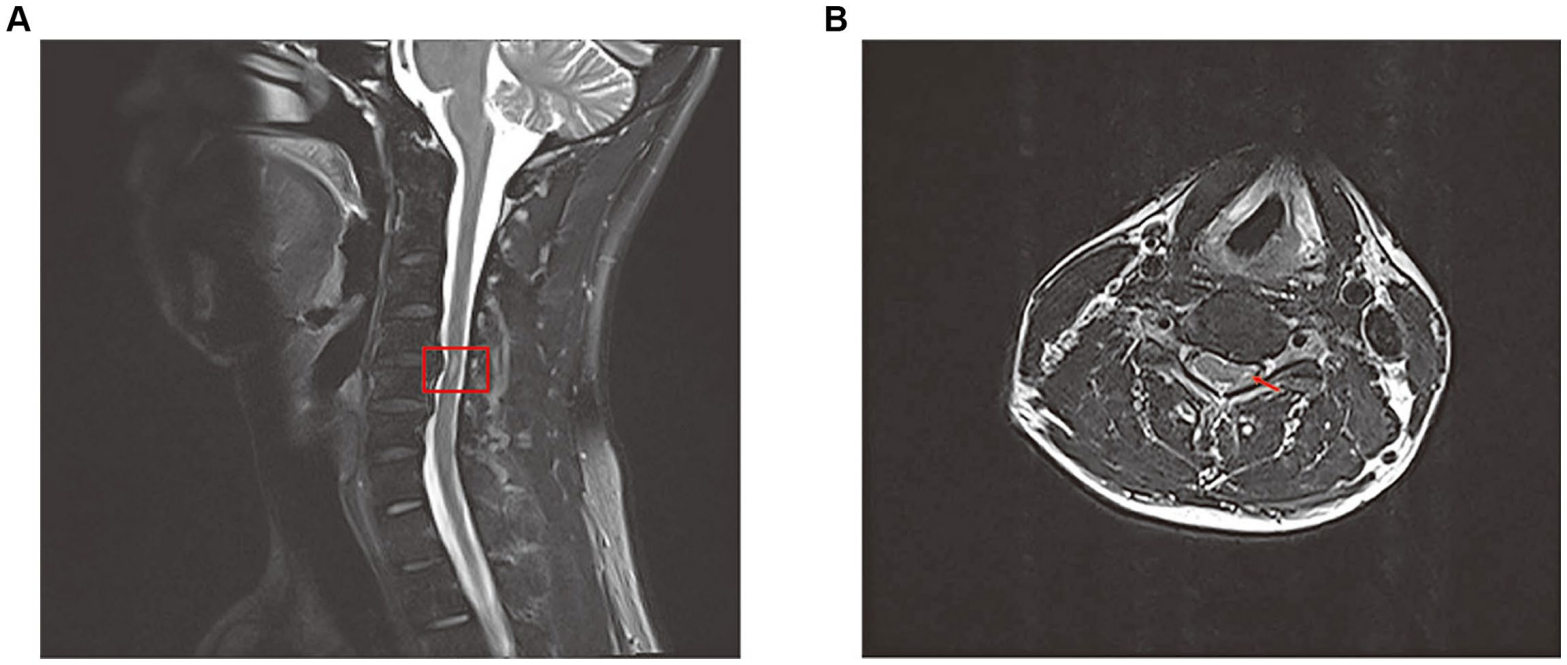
Total spine magnetic resonance image(MRI) revealed long strip T2 high signal in the left part of the cervical medulla at the level of the 4/5 intervertebral space on sagittal position (A) and cross section (B).
